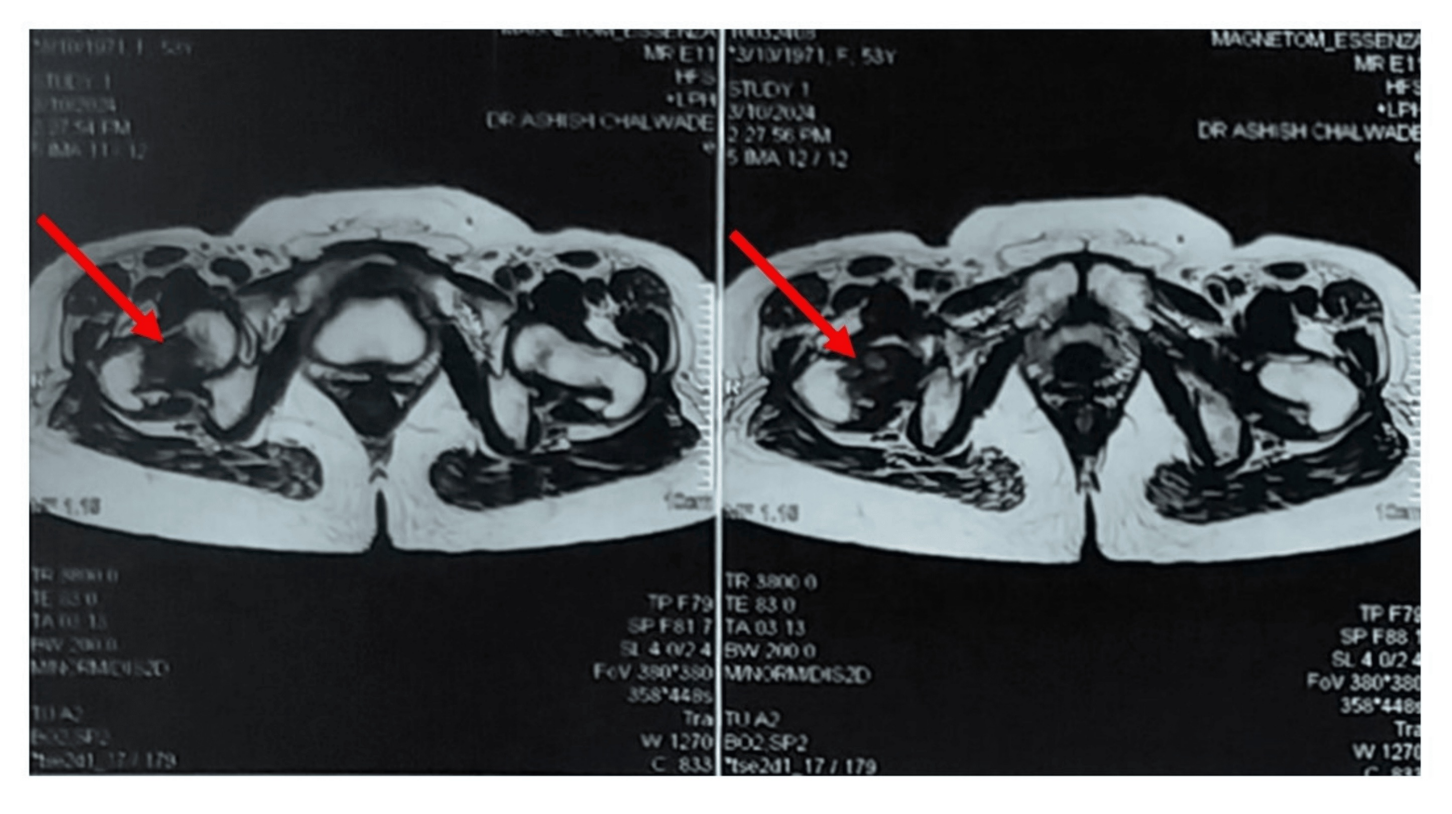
Axial MRI pelvis with both hips cuts showing proximal femoral metastasis The red arrow marks show a hypointense shadow in the axial cuts of the MRI, indicating the involvement of the neck of the femur and the intertrochanteric area.
